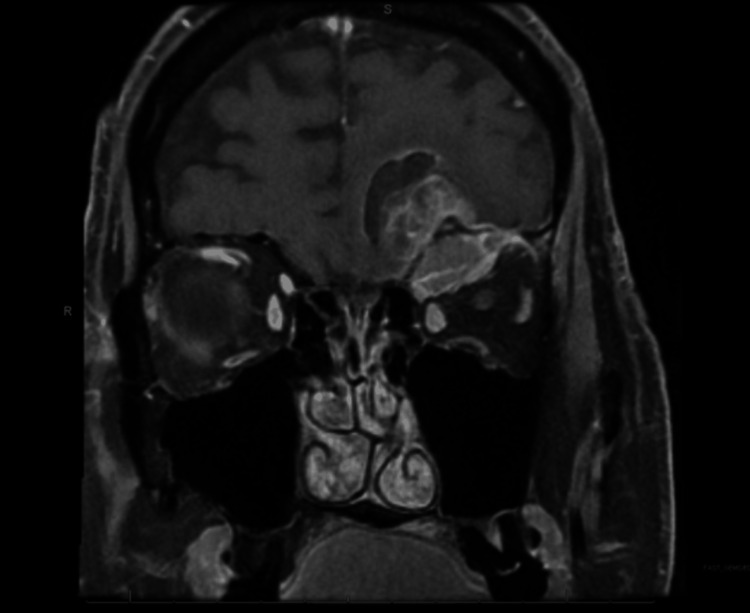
MRI brain and orbit coronal view
